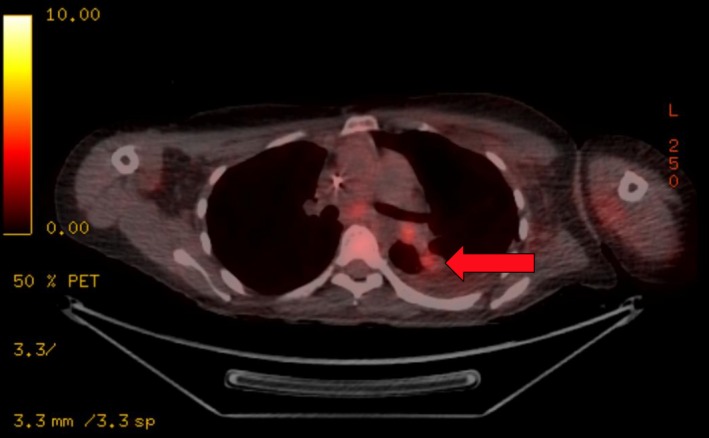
A PET‐CT scan showing FDG‐uptake into the pulmonary lesion (arrow), with associated lymphadenopathy
